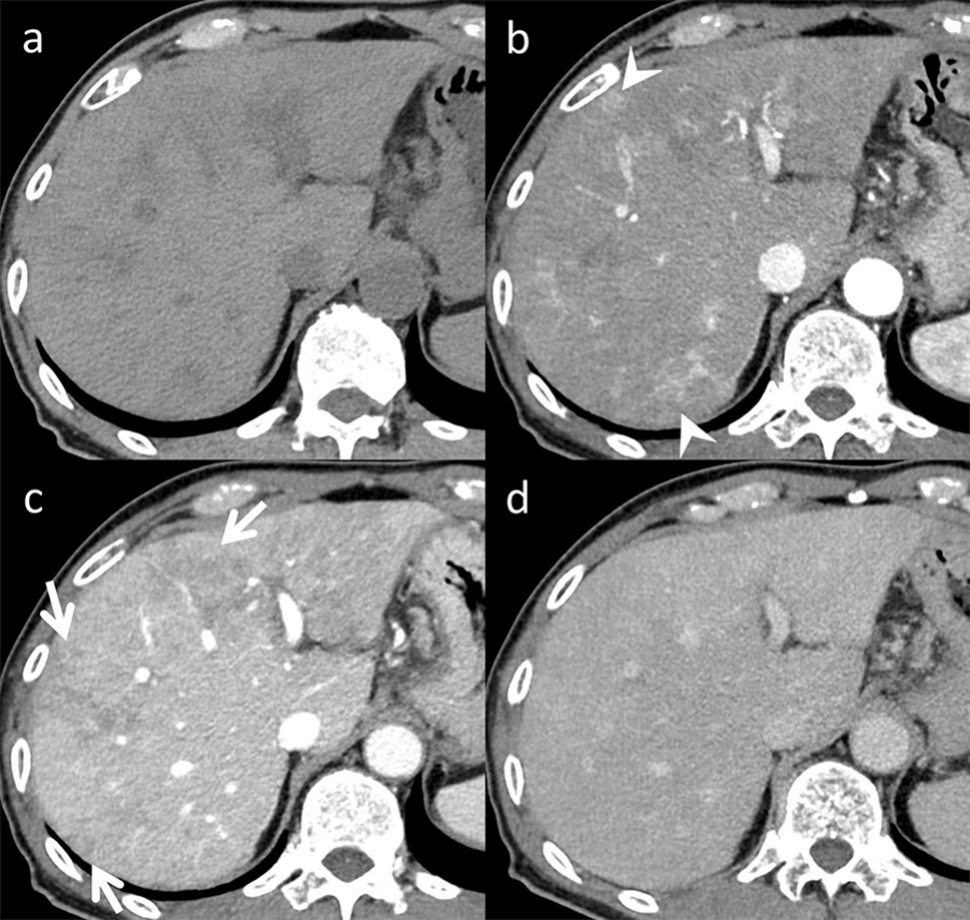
Sinusoidal obstruction syndrome in an asymptomatic 65-year-old man, who was undergoing chemotherapy (S-1 and oxaliplatin) for esophagogastric junction cancer. Dynamic computed tomography (CT) (a pre-contrast, b arterial phase, c portal venous phase, and d equilibrium phase) demonstrates diffuse reticular hypoattenuation in the liver, most obvious in the portal venous phase. These areas are unclear on pre-contrast and equilibrium phase images. Patchy heterogeneous enhancement is also observed in the arterial phase
